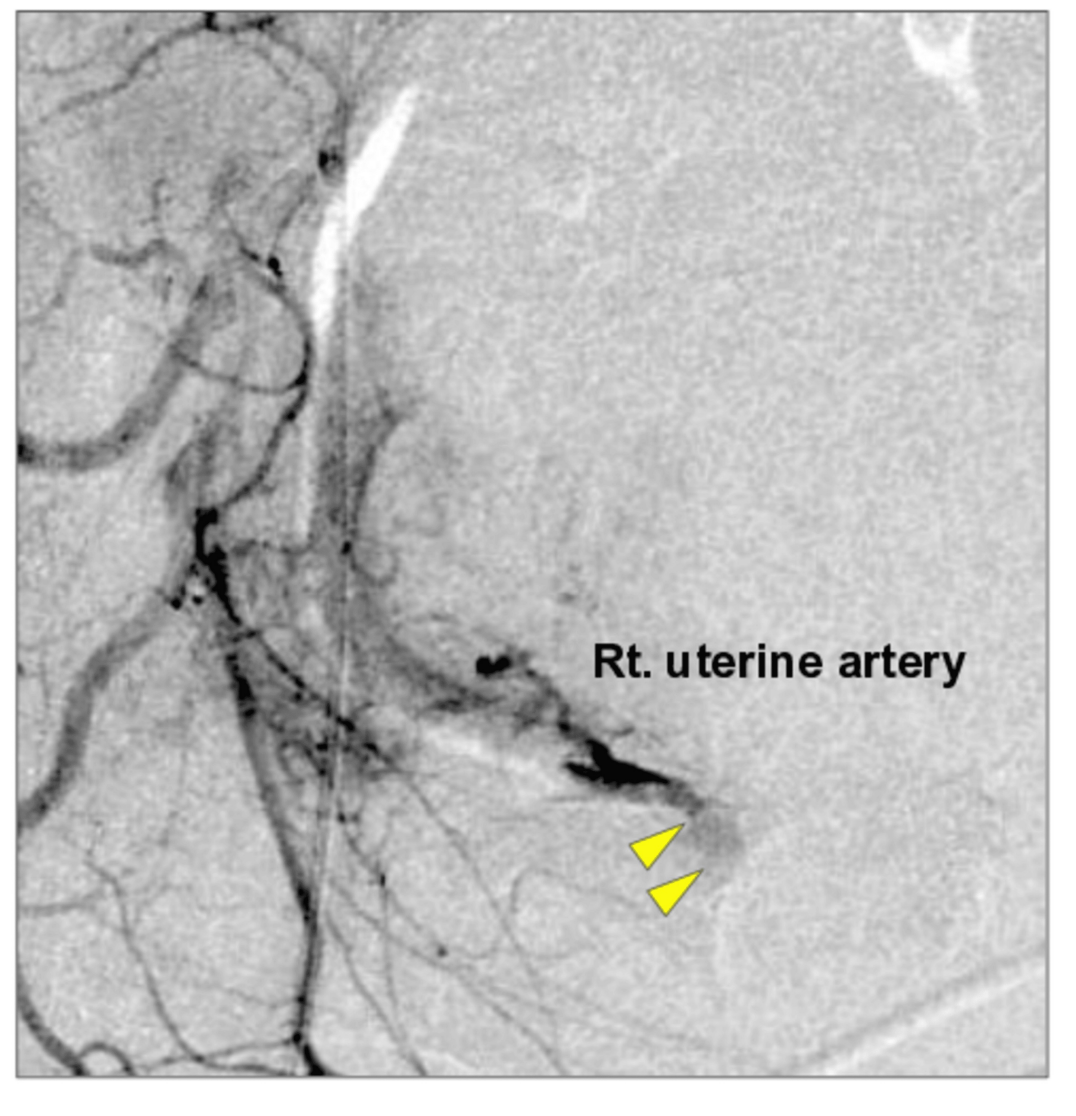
Delayed contrast extravasation requiring transcatheter arterial embolization (TAE). Follow-up contrast-enhanced computed tomography on postoperative day 18 demonstrating delayed-onset contrast extravasation from the right uterine artery (arrowhead). Emergency TAE was performed, achieving complete hemostasis.
